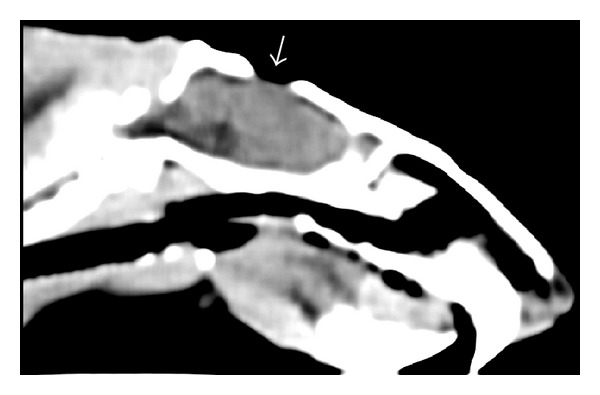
Visualization of 3D volumetric CT data sliced in sagittal plane using vendor supplied software. Arrow points to craniotomy.
